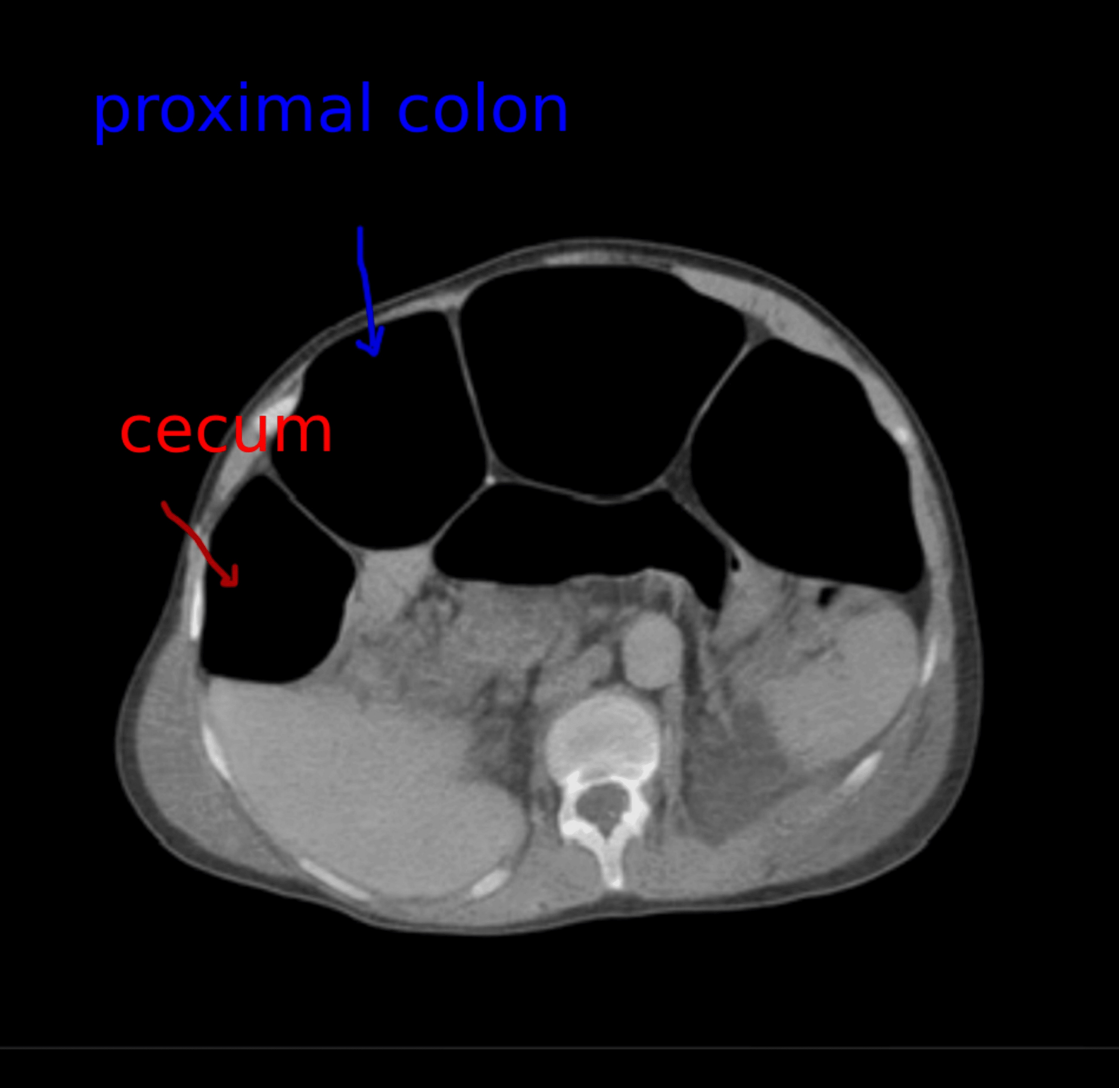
Axial contrast-enhanced CT of the abdomen showing marked gaseous distention of the cecum (red arrow) and proximal colon (blue arrow), measuring approximately 13 cm in diameter, without a transition point, consistent with acute colonic pseudo-obstruction (Ogilvie’s syndrome).
